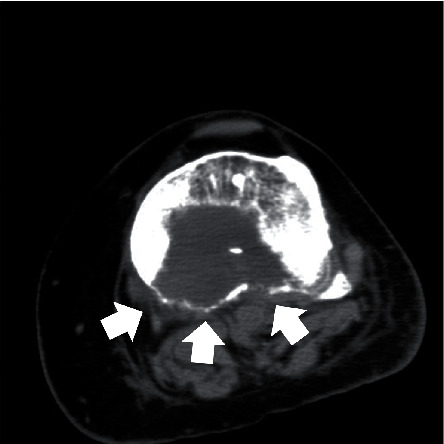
Preoperative axial CT-scan showing the destruction of the posterior cortex at the proximal part of the tibia (white arrows).
